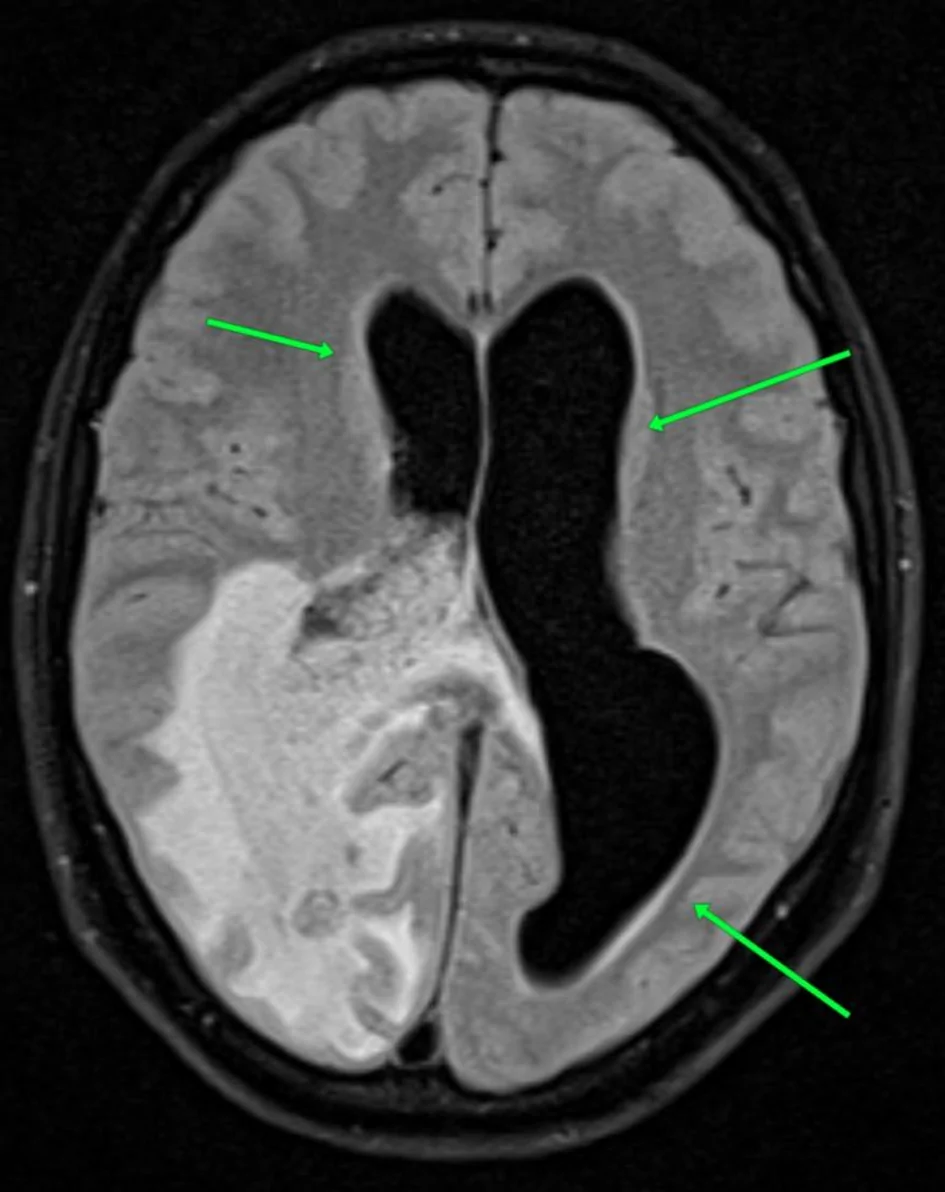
Axial T2-FLAIR MRI demonstrating moderate dilation of the lateral ventricles, more pronounced on the contralateral side (green arrows), consistent with obstructive hydrocephalus. FLAIR: fluid-attenuated inversion recovery; MRI: magnetic resonance imaging.
